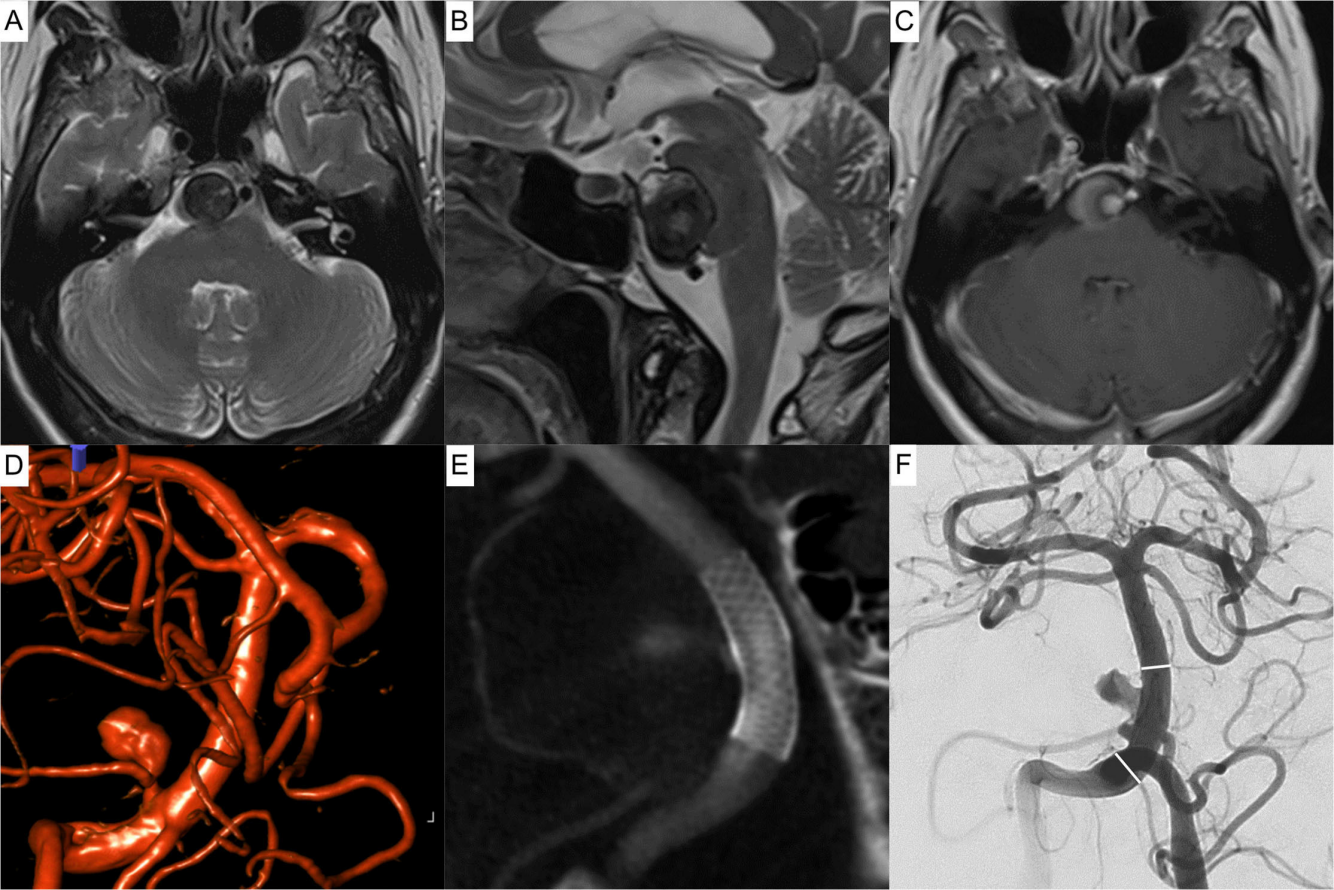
Indirect flow diversion for treatment of a partially thrombosed dissecting aneurysm of the right-hand side anterior inferior cerebellar artery in a 73-year-old patient. The perfused aneurysm (4.5 × 6.3 mm fundus, 2.0-mm neck) is 2.4 mm distant to the basilar artery. Upper row (A–C) magnetic resonance imaging of the incidental aneurysm compressing the pons. (A) Axial T2 weighted image showing the partially thrombosed aneurysm of the pre-meatal segment of the anterior inferior cerebellar artery lateral to the basilar artery. (B) Corresponding sagittal T2 weighted image demonstrating the mass effect of the aneurysm. (C) Axial T1 weighted image post Gadolinium showing the basilar artery, the perfused part of the aneurysm and the thrombosed portion. Middle row (D–F): Peri-interventional images. (D) Reconstruction of a 3D angiogram prior to treatment, showing the relationship between basilar artery, parent vessel, and aneurysm. (E) Contrast enhanced Xper-CT after implantation of a PED Flex Shield 4.75 × 14 mm into the basilar artery, jailing the aneurysm, bearing anterior-inferior cerebellar artery. (F) Postinterventional angiogram: aneurysmal perfusion is immediately altered (O'Kelly-Marotta Grade A3). The white lines indicate the proximal and distal endings of the implanted device. At the last available imaging study, 3 months post implantation, the aneurysm discretely decreased in size (not shown).
